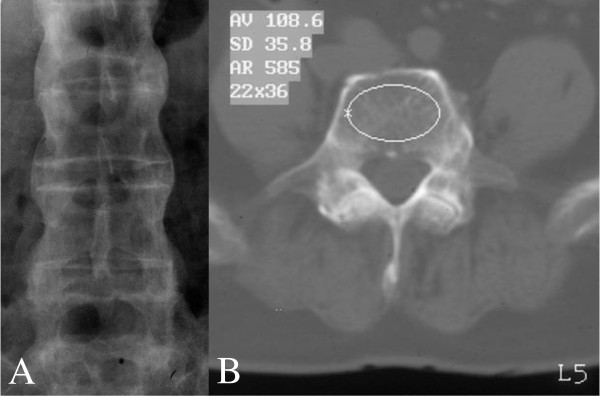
(A) Anteroposterior lumbar radiograph with advanced syndesmophytes and (B) Quantitative Computed Tomography scan of L5 vertebral body showing elliptical region of interest (ROI) containing trabecular bone (48-year-old patient at baseline).
